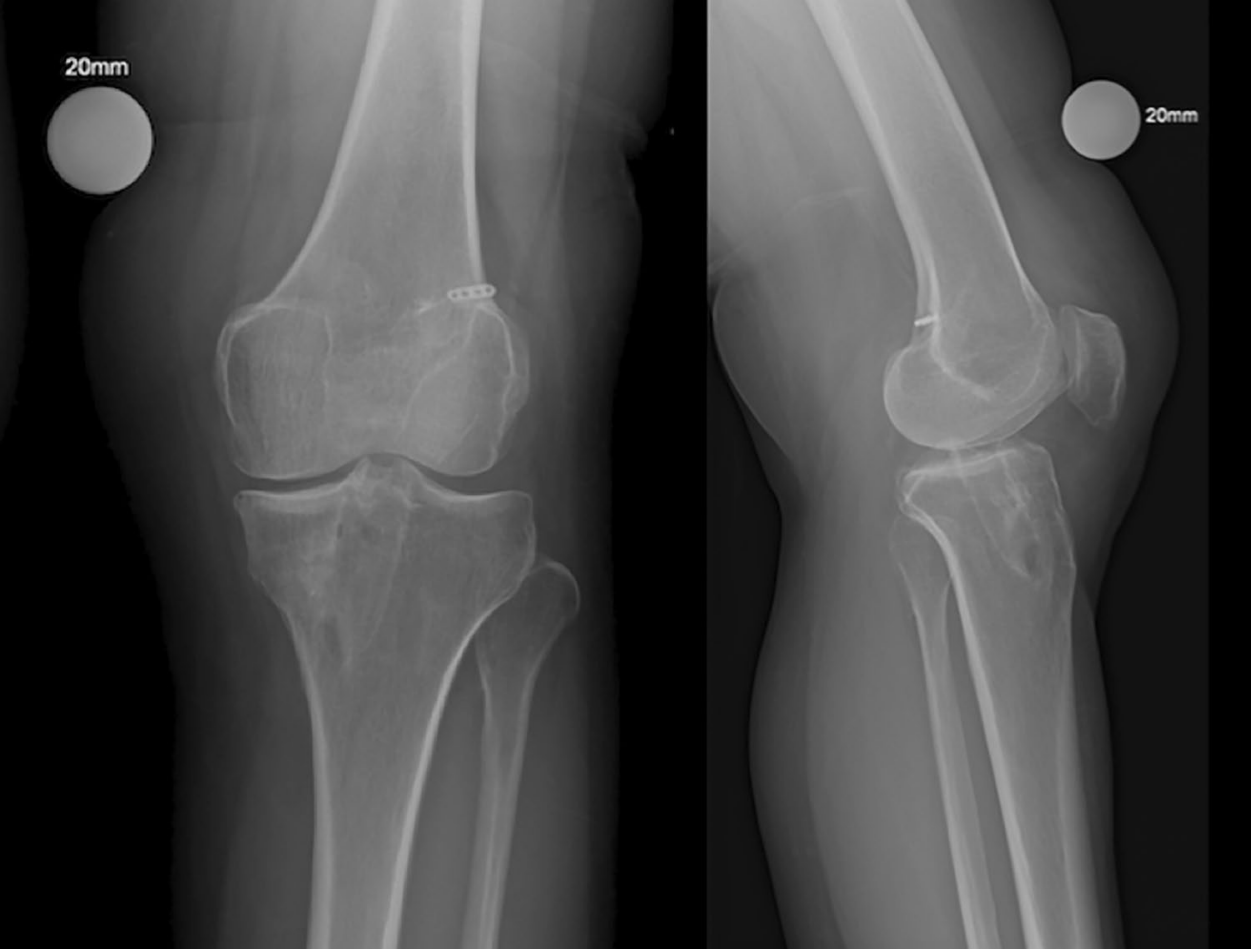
Pre-operative anteroposterior and lateral X-ray of a re-revision surgery case; left knee. The widened and increased tibial tunnel and probable mobilisation of the femoral plateau are visible
